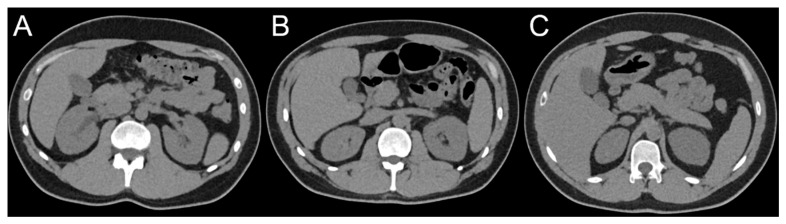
Examples of CT scans using the image quality levels (IQLs) 70 (A), 60 (B) and 50 (C).
